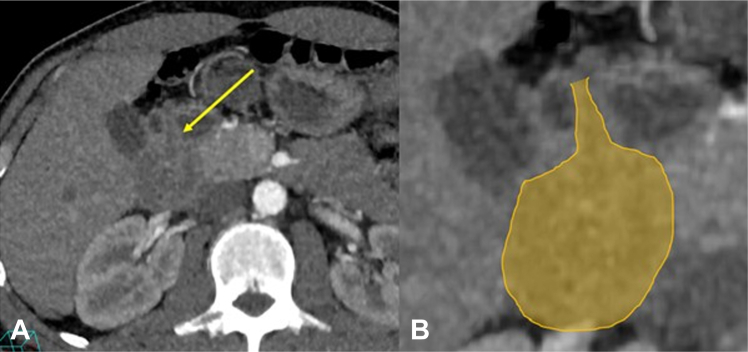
A, Arterial phase CT axial image showing a large filling defect distending the duodenum (*arrow*). B, Magnified view showing the lesion in *yellow color*.
